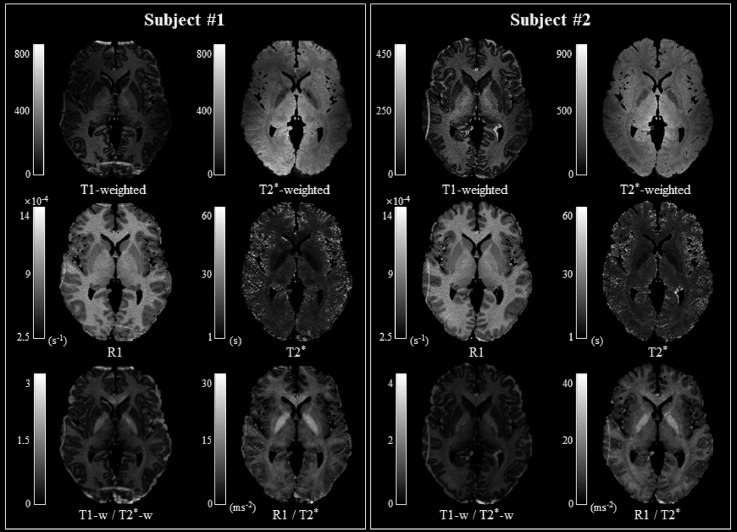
Comparison of myelin-related mapping methods. The myelin-related maps obtained with the T1-weighted/T2*-weighted (w-Ratio) and R1/T2* (q-Ratio) methods are similar in both subjects. Note that the FOV was not optimized during image acquisition and that aliasing artifacts occurred, depending on the subjects’ head sizes, mainly along the anterior and posterior regions in Subject 1 and the left and right regions in Subject 2. Abbreviations: FOV, field-of-view.
